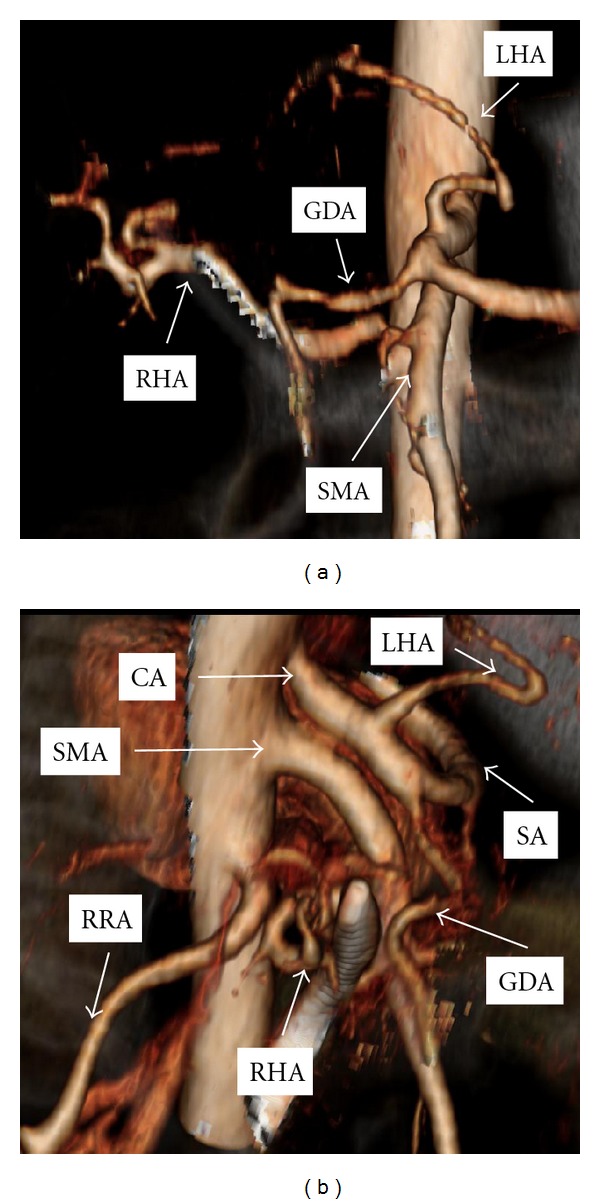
Coronal (a) and lateral (b) computed tomography reconstruction of mesenteric arterial vasculature in Case 1. Structure overlying right hepatic artery represents common bile duct stent. GDA: gastroduodenal artery, LHA: left hepatic artery, RHA: right hepatic artery, SMA: superior mesenteric artery, CA: celiac axis, RRA: right renal artery, SA: splenic artery.
